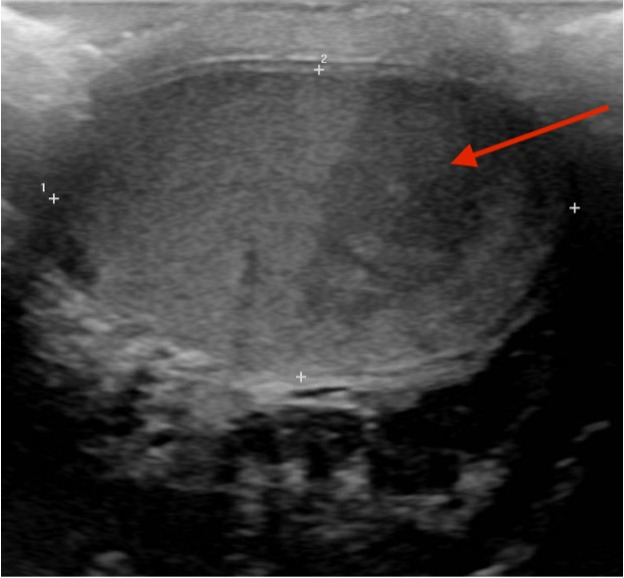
A hypoechoic wedge-shaped area (arrow) seen on ultrasound in the right
